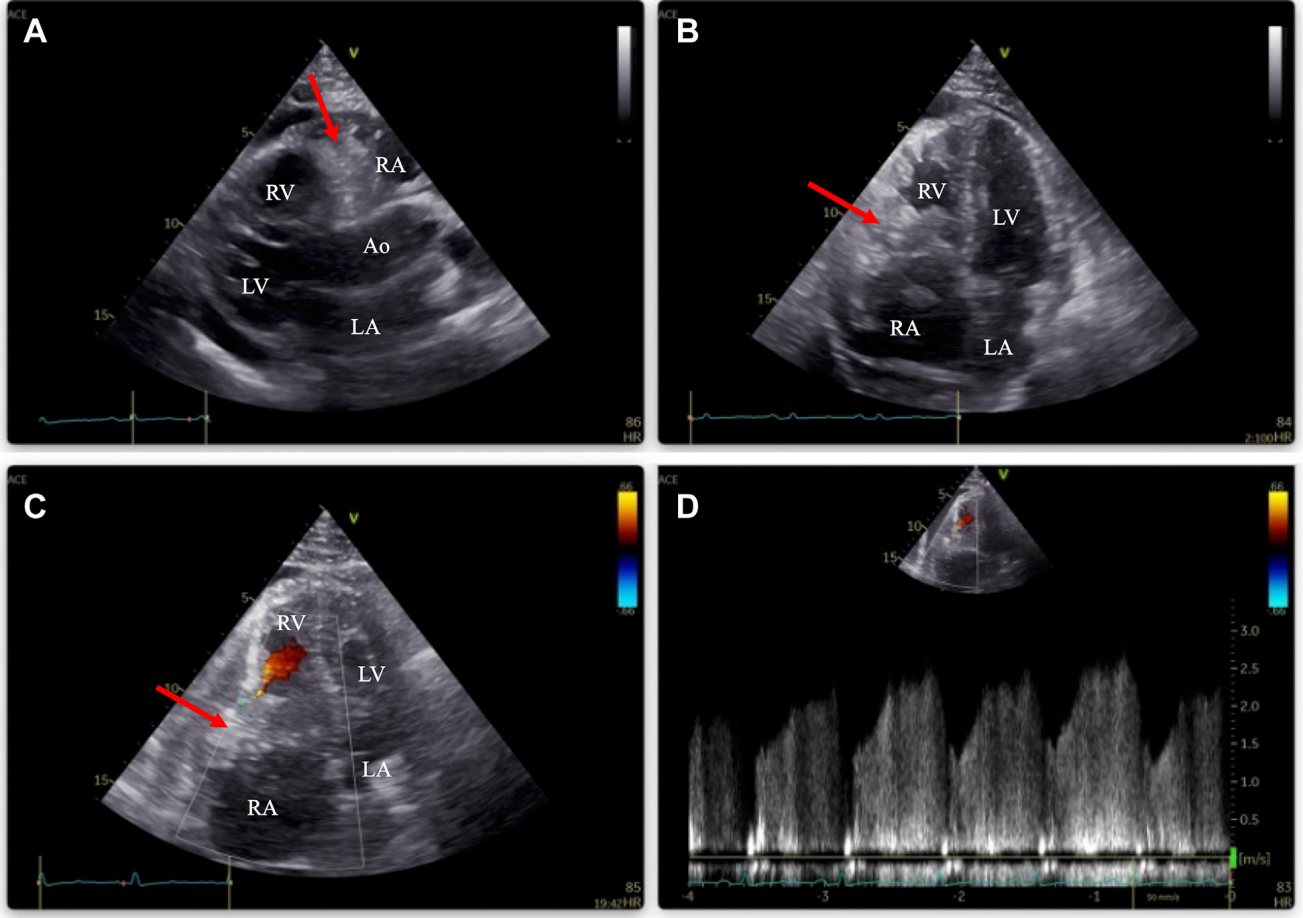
Transthoracic Echocardiography (A) Parasternal long-axis view and (B) 4-chamber view indicate that the mass (red arrows) is almost stuck in the tricuspid annulus. (C) Color Doppler image: Just a little inflow is observed. (D) Transtricuspid flow is continuous. The estimated diastolic pressure gradient of the tricuspid valve is 23 mm Hg. Ao = aorta; LA = left atrium; LV = left ventricle; RA = right atrium; RV = right ventricle.
